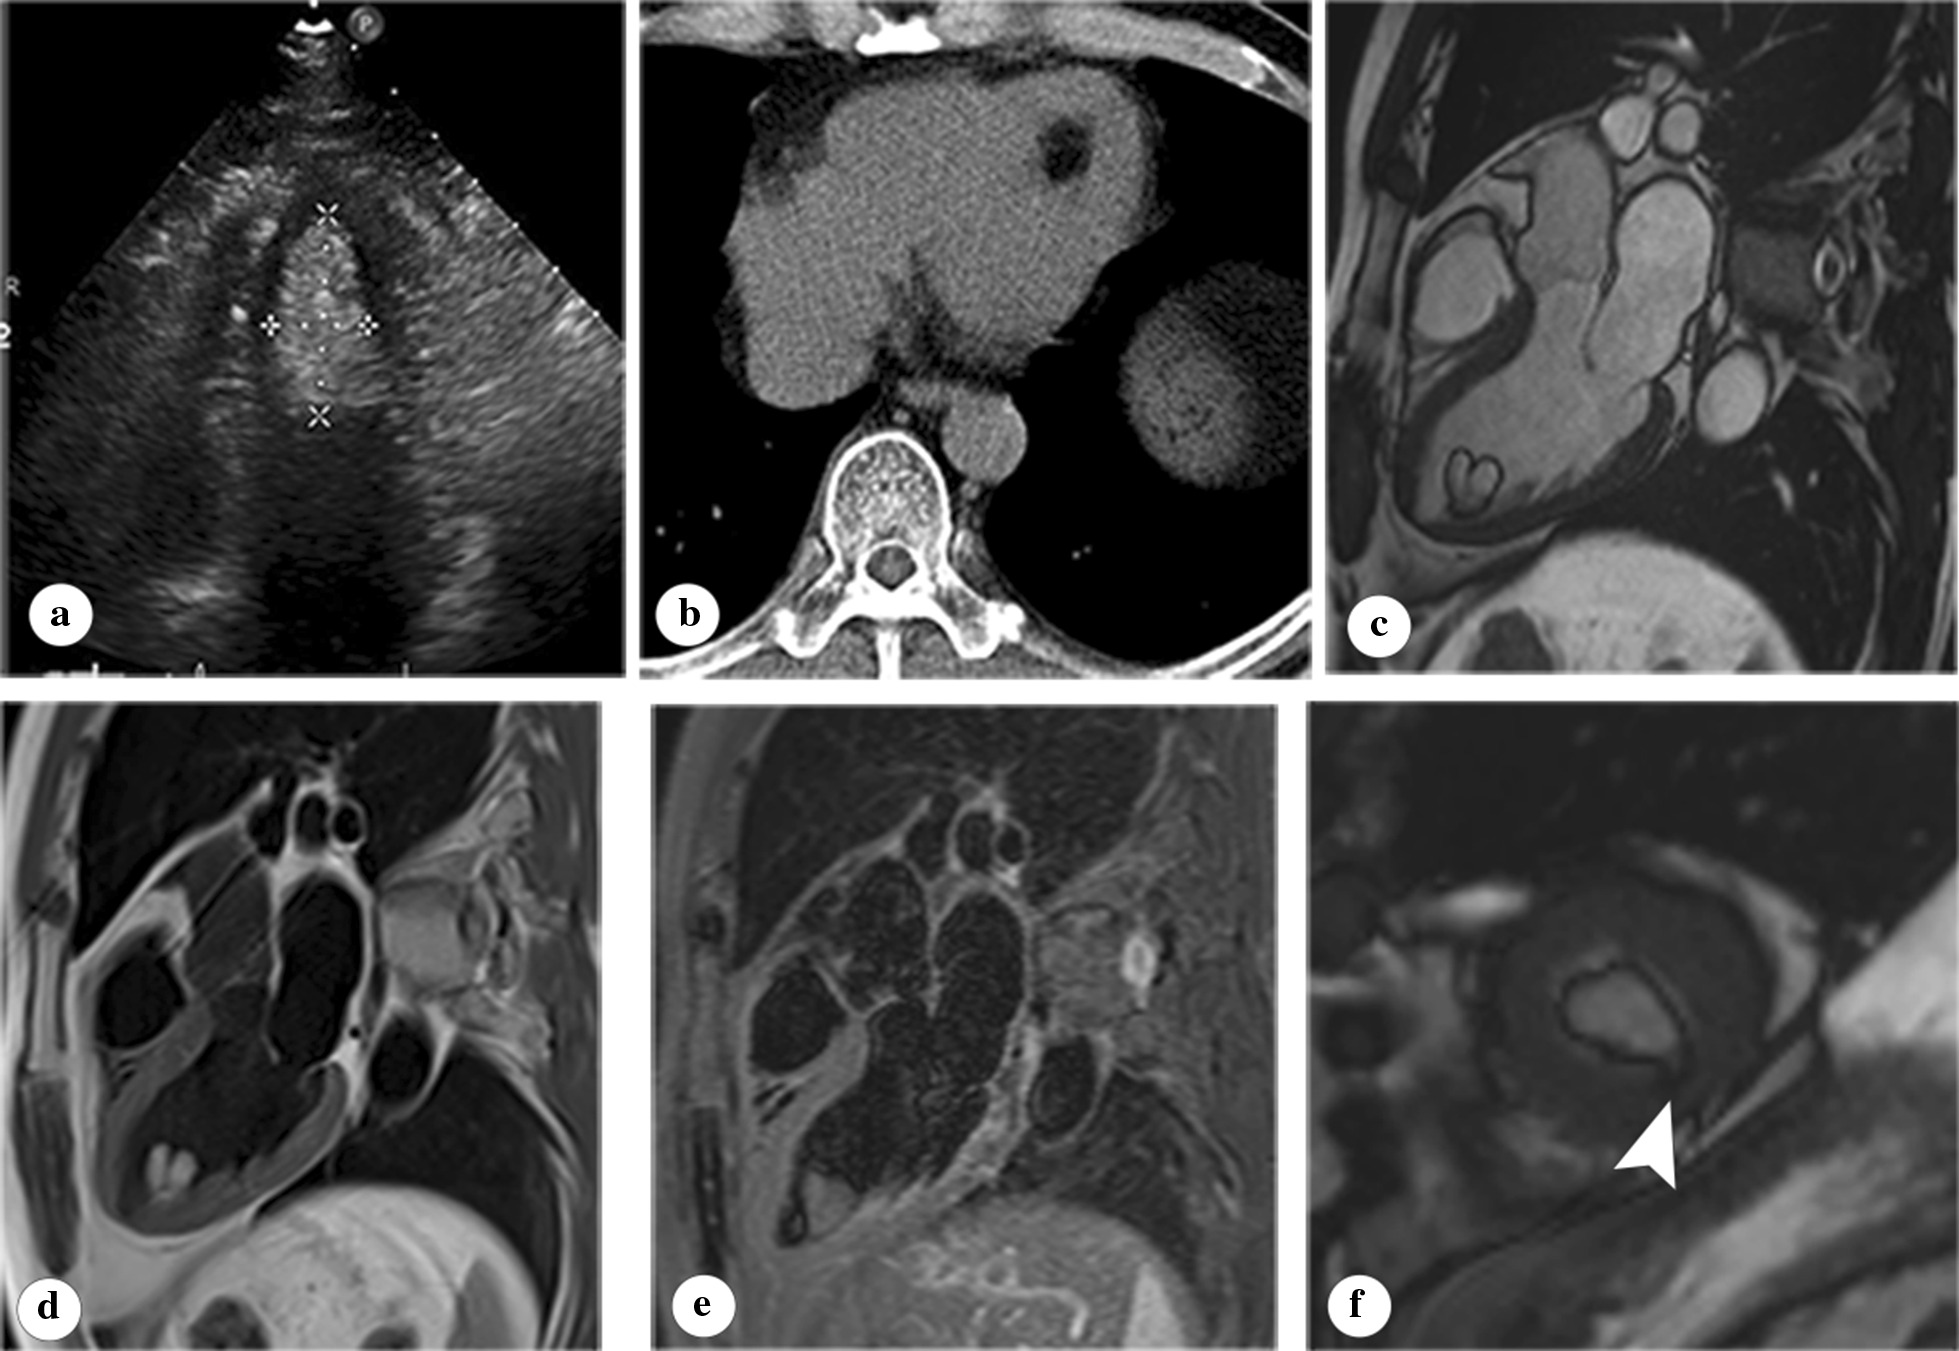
Images of a ventricular lipoma in an asymptomatic male patient (part of pictures have been previously published in the review by our griup [13]). The ventricular lipoma presented as a well-defined homogenous hyperechoic mass within left ventricle (a). The lipomas showed homogenous low density (-100 HU) on CT (b). On cardiac MRI, the lovely heart-shaped lipoma showed same signal intensity with subcutaneous fat in all sequences including cine sequence (c), T1 weighted image (d), T2 weight fat suppression image (e). Particularly, the narrow pedicle of the lipoma was depicted in short axis image attached to the endomyocardium (f, arrowhead)
